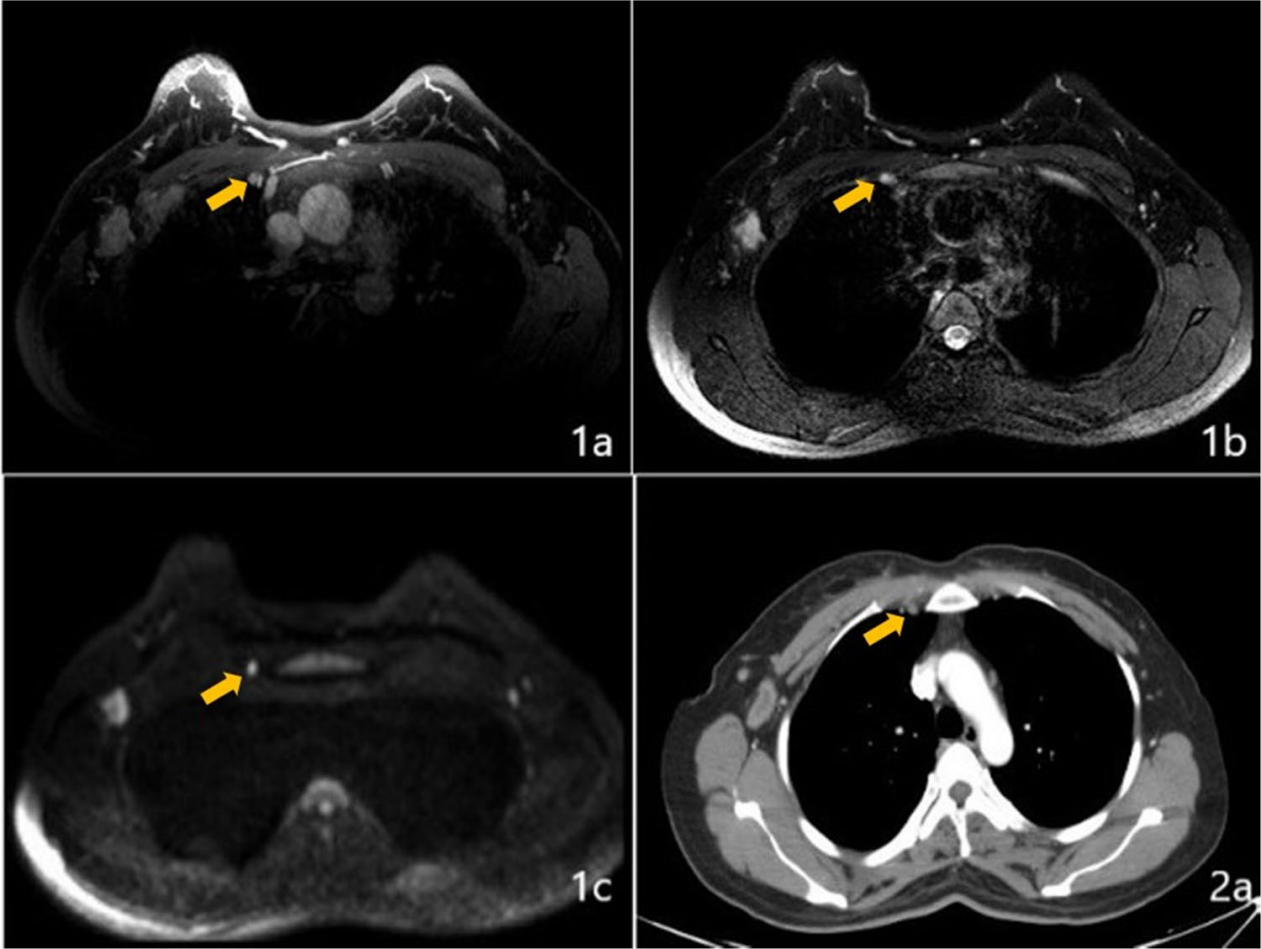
A metastatic internal mammary lymph node (yellow arrow) detected during using dynamic contrast-enhanced T1-weighted magnetic resonance imaging (1a), T2-weighted imaging (1b), diffusion-weighted imaging (1c), and computed tomography (2a)
